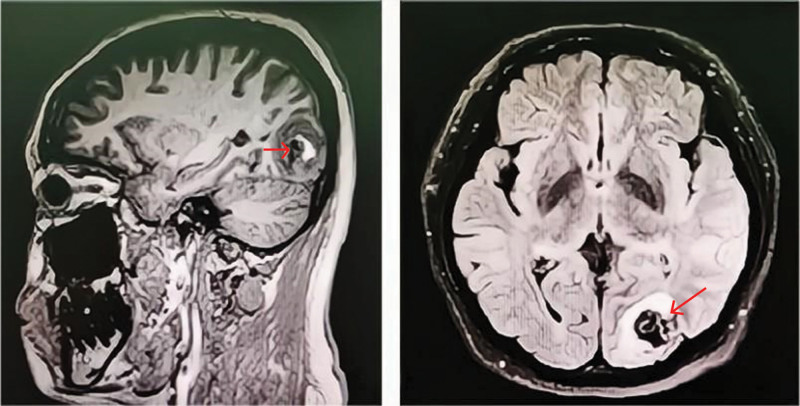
MRI of patient’s brain shows the lesion of AVM (arrow). AVM = arteriovenous malformation.
